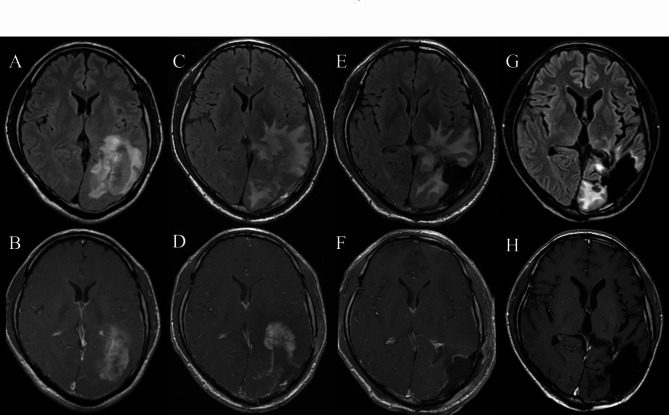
Summary of magnetic resonance imaging axial images. Preoperative magnetic resonance imaging (MRI) reveals a heterogeneously gadolinium-enhanced lesion with edema in the left temporo-occipital lobe (A: FLAIR, B: contrast-enhanced T1). MRI before the second surgery shows a contrast-enhanced lesion with edema deep in the postoperative cavity (C: FLAIR, D: contrast-enhanced T1). MRI after the second surgery shows that all contrast-enhanced lesions have been resected (E: FLAIR, F: contrast-enhanced T1). The final MRI, performed 37 months after the initial surgery, shows no intracranial tumors (G: FLAIR, H: contrast-enhanced T1)
